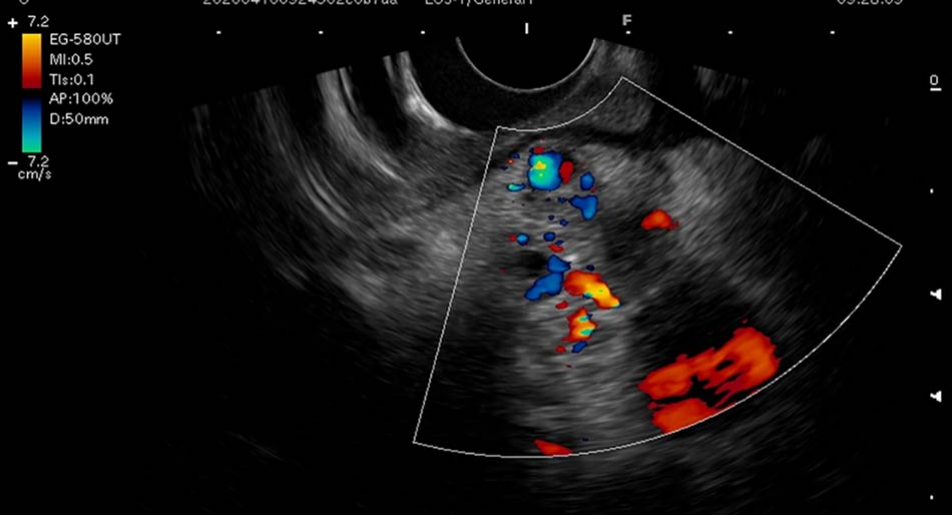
Endoscopic ultrasonography image. Endoscopic ultrasonography showed tortuous vessels and low echoic lesions in the pancreas head, and no findings suspicious of malignancy.
